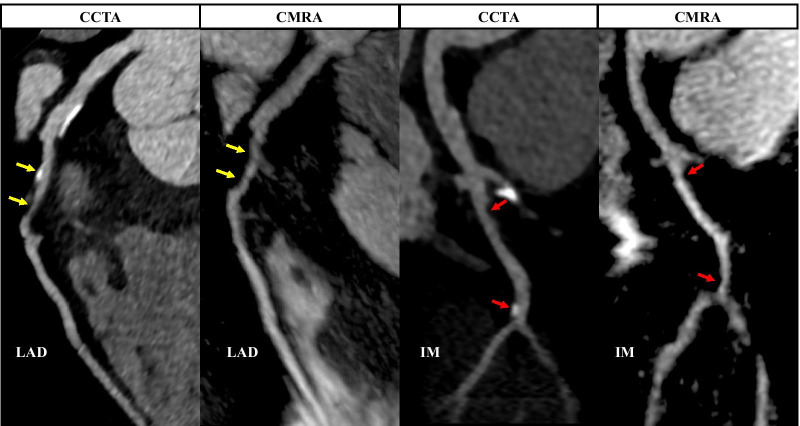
Curved multiplanar reformat non-contrast coronary CMRA and contrast enhanced coronary CTA in a 57 year old male with > 50% partially calcified stenosis in the proximal LAD (yellow arrows). The red arrows represent focal < 50% stenosis in the proximal and distal ramus intermedius artery. IM-Ramus intermedius artery
